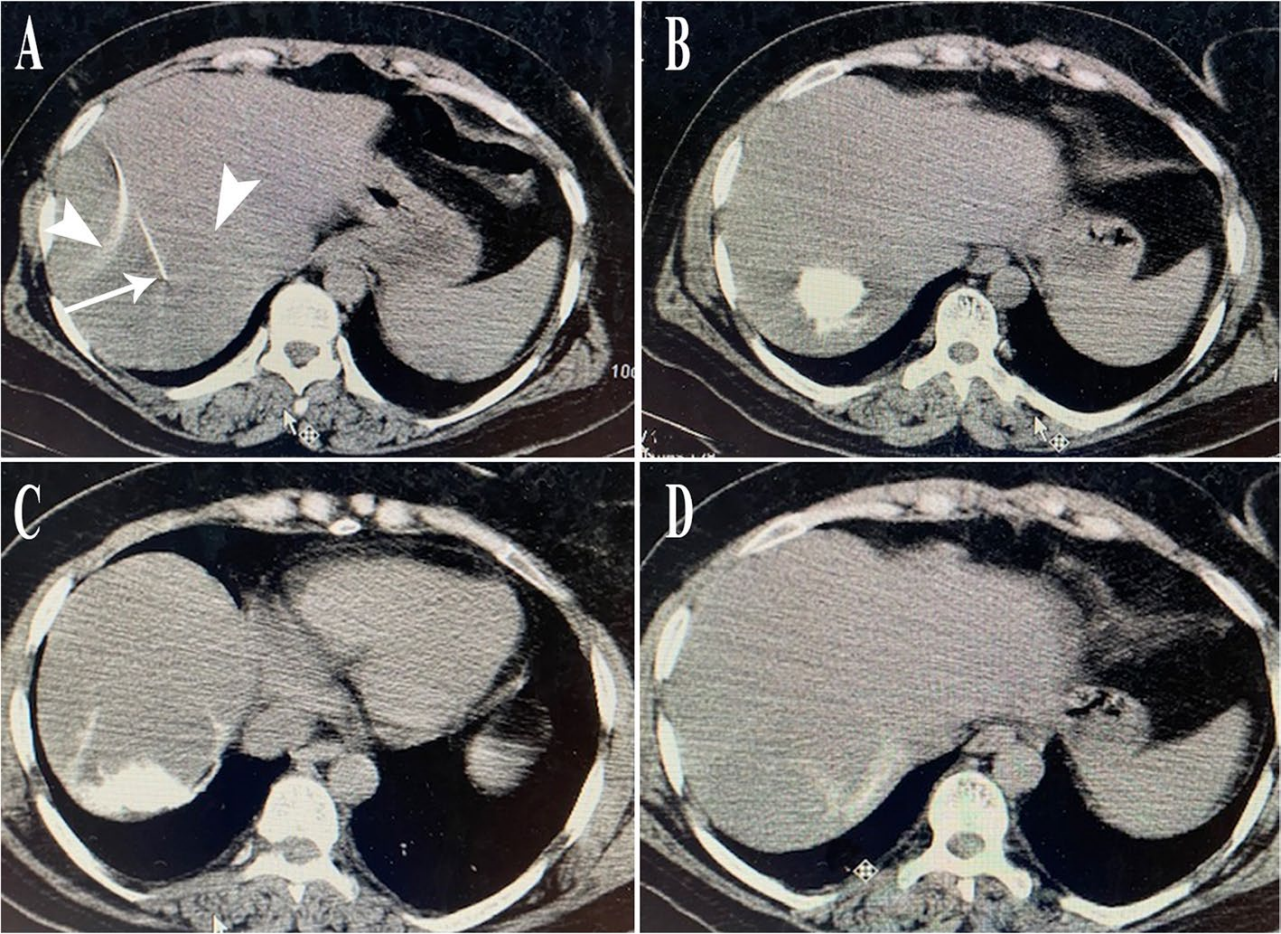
A Hepatic venous malformation (arrowheads) in the posterior right lobe of a middle-aged woman is accessed with a 22-gauge Chiba needle under CT guidance. The tip of the needle is marked with an arrow. B Water-soluble contrast is injected into the lesion. C, D Slow infusion of bleomycin solution shows the dissemination of contrast/bleomycin mixture within the lesion after 2 and 4 min, respectively
